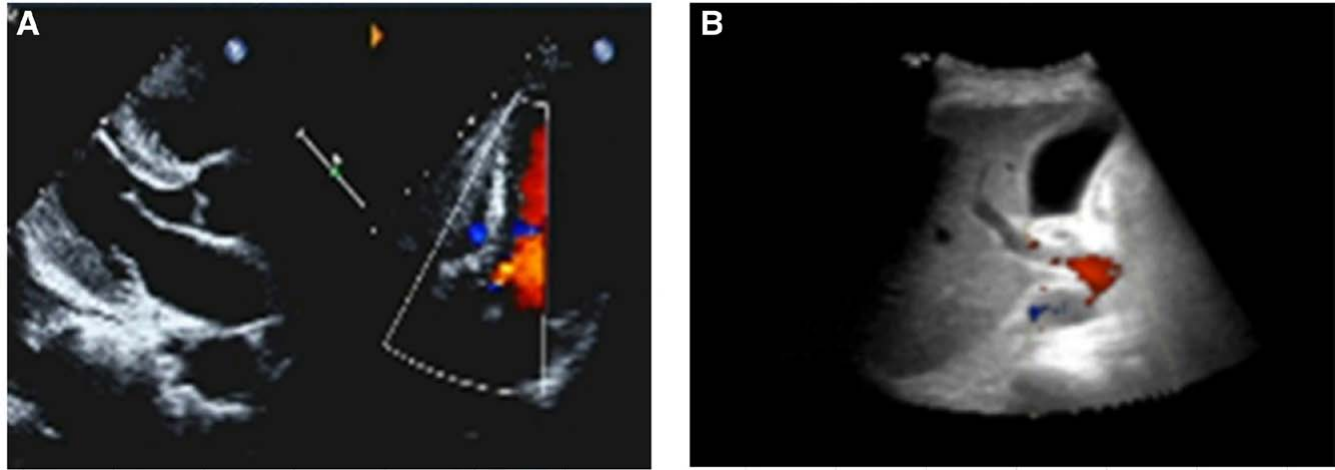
Changes in cardiac ultrasound and abdominal ultrasound during patient admission. (A) Changes of Doppler echocardiography at admission. (B) Changes of abdominal ultrasound at admission.
